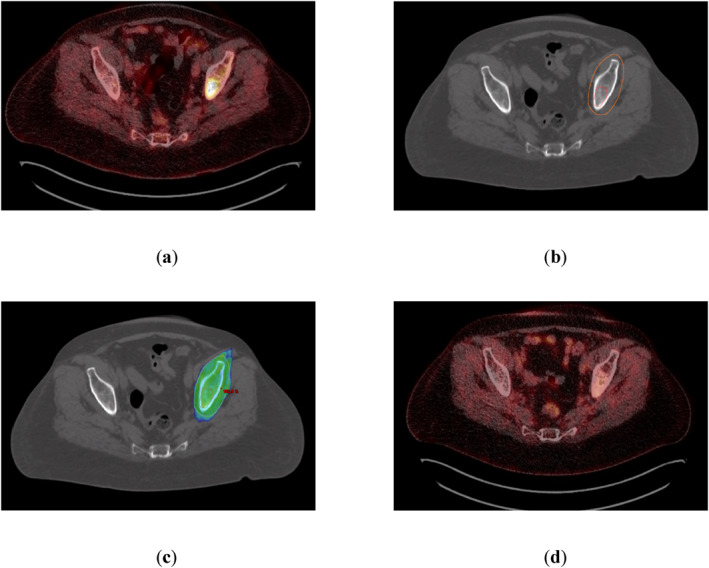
Salvage radiotherapy (RT) for a 61‐year‐old woman presenting with oligoprogressive disease in the left iliac bone on a Positron emission tomography‐computer tomography (PET‐CT) scan 30 days after chimeric antigen receptor T‐cell (CAR‐T) therapy. No more lesions were detected. Serological the patient showed a very good partial response. Interdisciplinary tumor board voted for local RT. (a) PET‐CT scan performed 30 days after CAR‐T therapy reveals a hypermetabolic lesion in the left iliac bone (standard uptake value 15; Deauville‐Score 5). (b) Planning‐CT indicating the PET‐CT‐based Gross Tumor Volume (red) and Planning Target Volume (orange). (c) 95%‐isodose as color‐wash dose distribution based on a 15 MV intensity‐modulated‐radiotherapy‐plan using 5 fields (prescribed dose 35 Gy in fractions of 2.5 Gy) (d) PET‐CT scan performed 30 days after completion of RT showing a complete metabolic remission.
